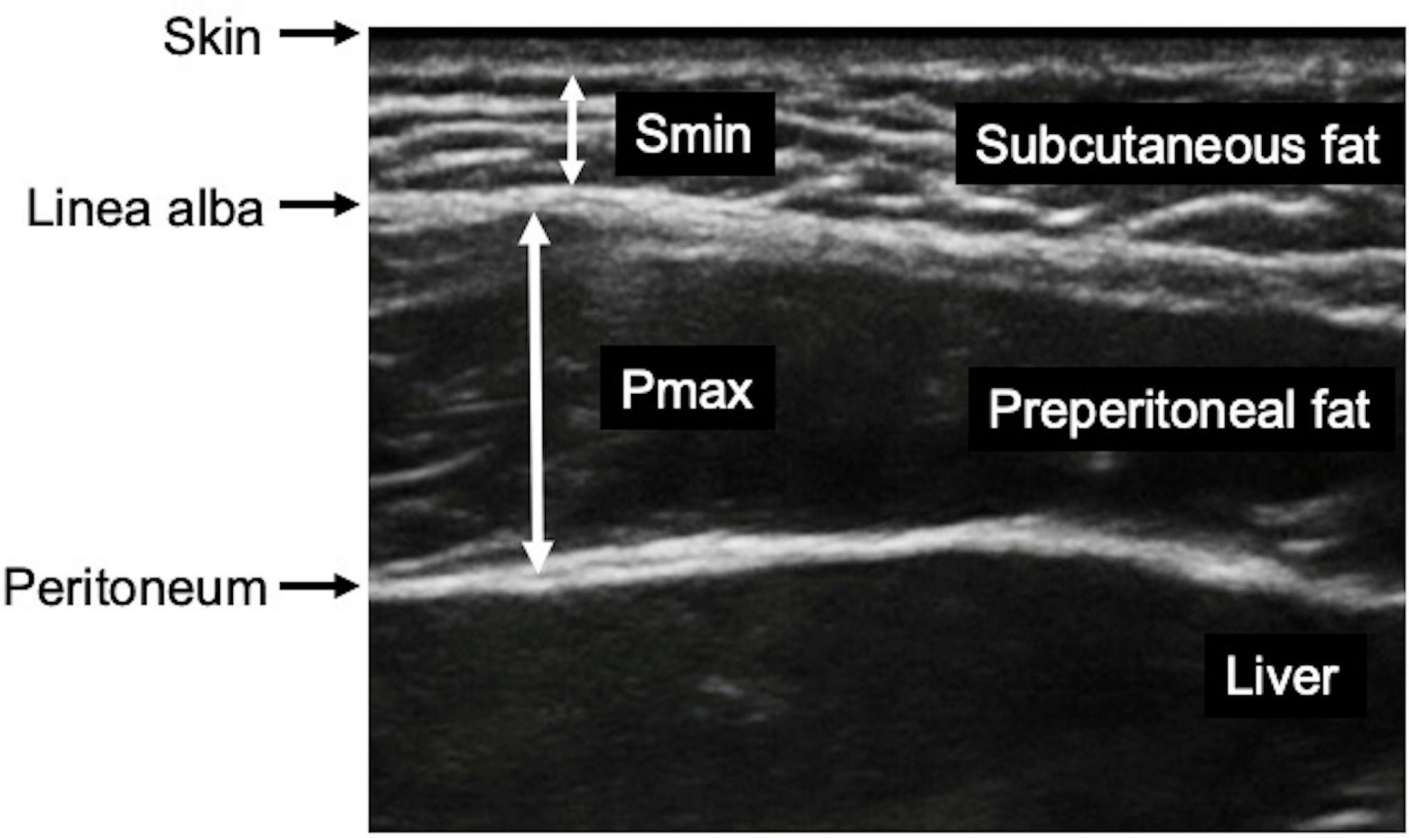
Ultrasound image of the upper abdominal wall on the xiphoumbilical line. Electronic calipers were used to measure the maximum preperitoneal fat thickness (Pmax) and minimum subcutaneous fat thickness (Smin).
